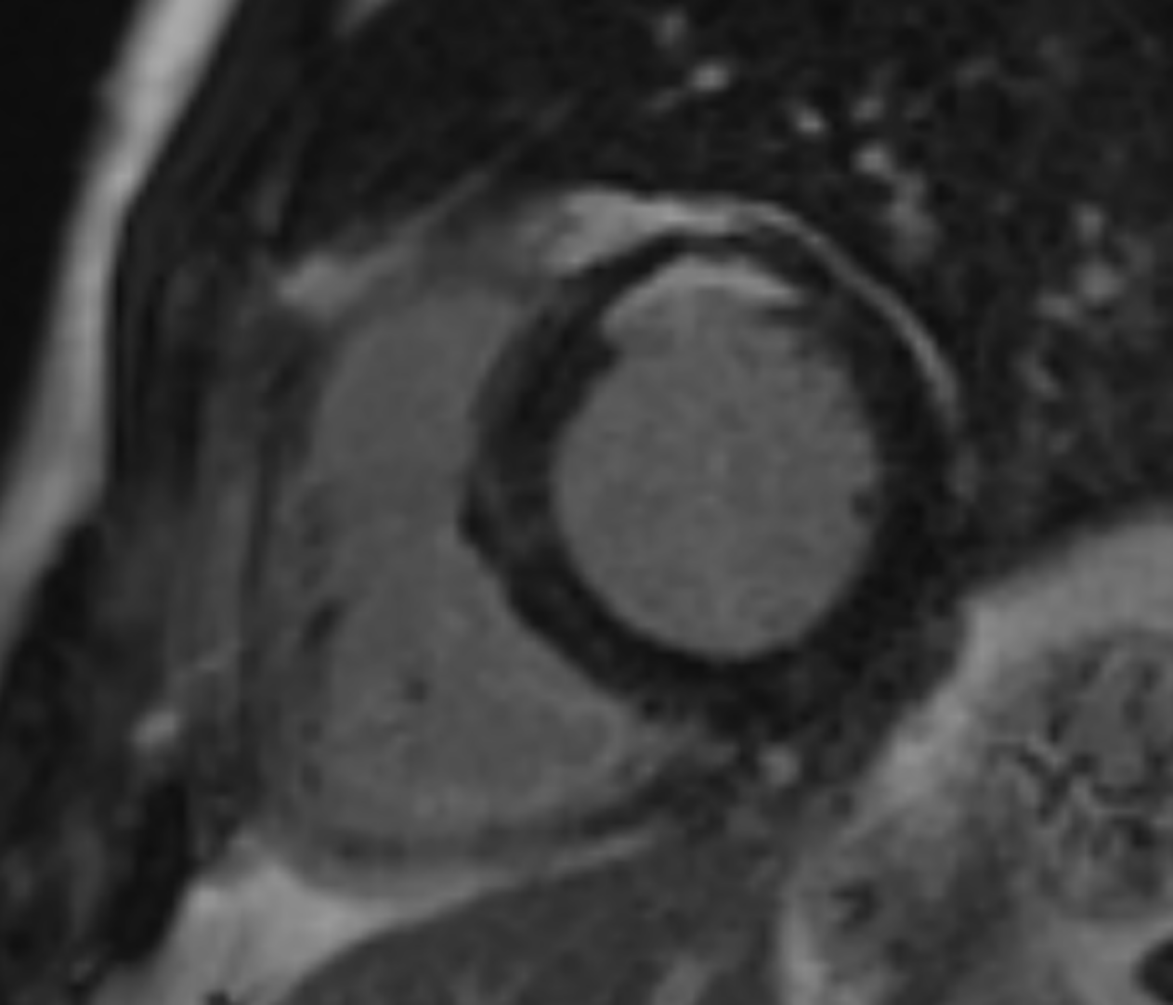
Unrecognized myocardial infarction as detected with cardiac magnetic resonance imaging in a 58-year-old male. Late gadolinium enhancement image demonstrated subendocardial hyperenhancement at anterior wall of left ventricular base level. His coronary artery calcium score was 462.
